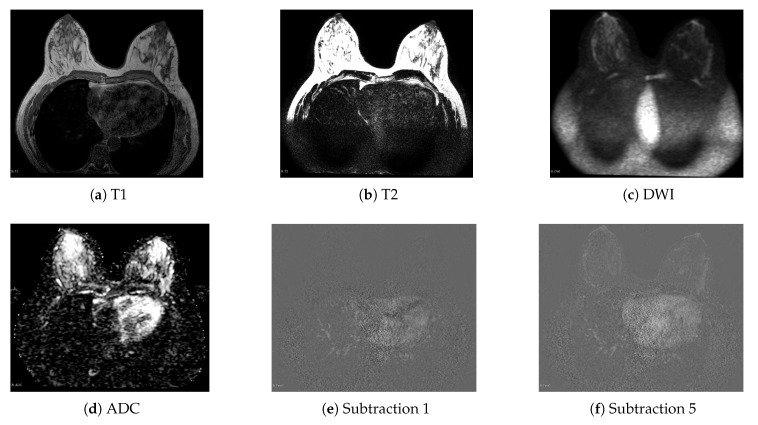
Visualization of the MRI sequences in the breast cancer dataset.
